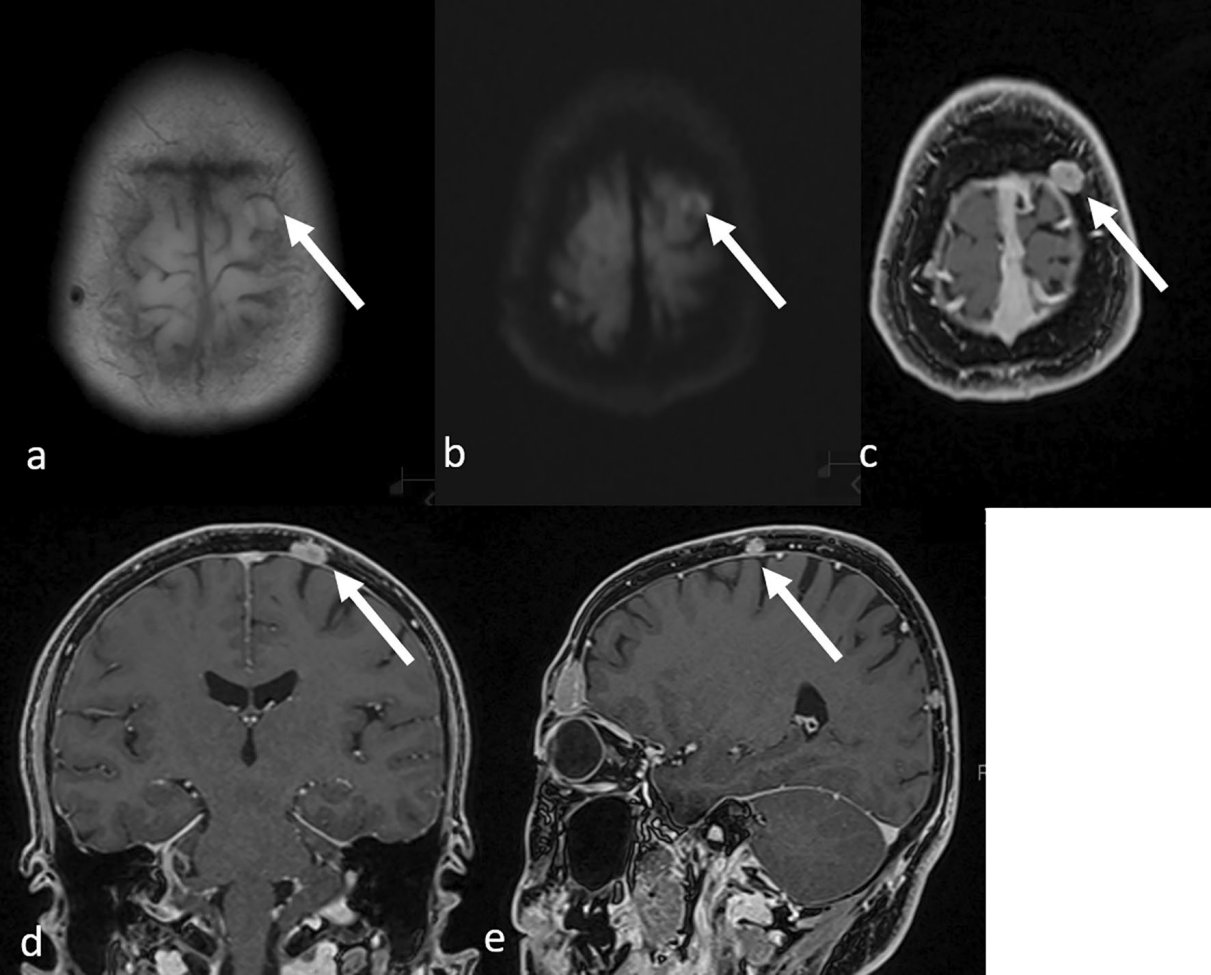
Osteolytic metastases on top of the left parietal bone in a patient with breast cancer. T1-weighted a and diffusion-weighted b images do not clearly show the tumor (arrows), whereas contrast-enhanced 3D fat-suppressed T1-weighted images with multiplanar reconstruction (MPR) in the axial c, coronal d, and sagittal e views clearly show the lesion (arrows)
